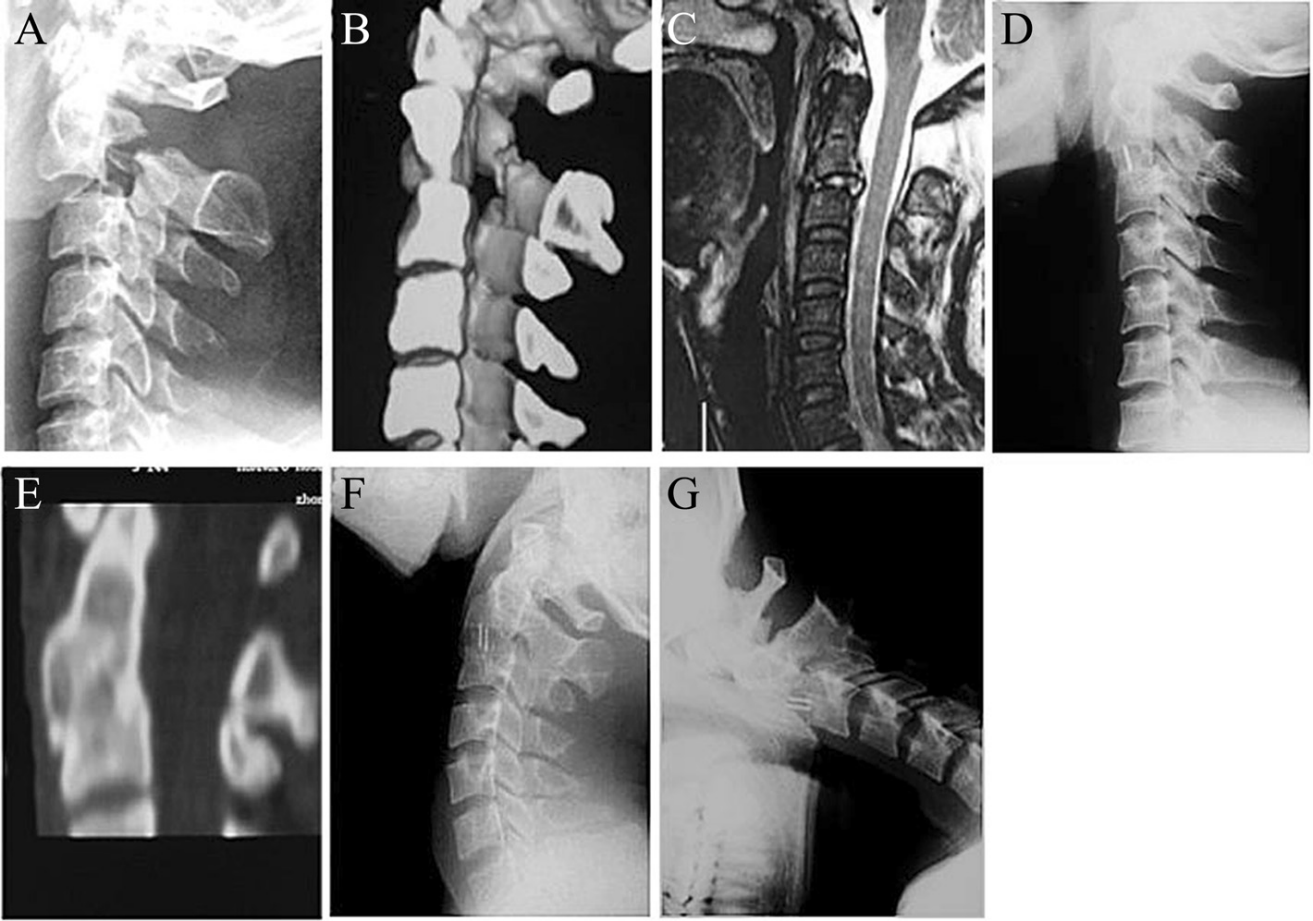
Images of a 40-year-old male patient. a and b, preoperative lateral X-ray and CT scans showing a type II Hangman’s fracture with severe translation. c, MRI with sagittal section showing C2/3 intervertebral disc injury. d, 3-month postoperative lateral X-ray showing adequate reduction and bony fusion. e, CT with sagittal reconstruction showing solid fusion and fracture healing. f and g, 24-month flexion/extension lateral X-rays showing no range of motion at the fusion site
